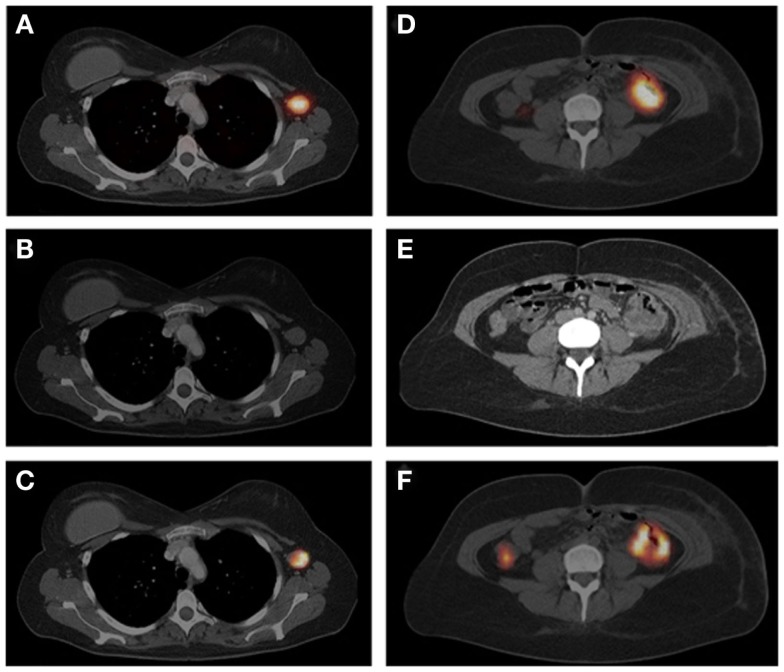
The SPECT/CT image (A), acquired 24 h after injection of ^111^In-IMP288 (185 MBq, 25 μg), pretargeted with 75 mg TF2 (1-day interval), in a 38-year-old patient (cohort 4), shows very clear tumor targeting of an axillary lymph-node metastasis, with very low concentrations of radioactivity in normal tissues. Corresponding contrast-enhanced CT scan and a fused FDG-PET/CT scan are shown [(B,C), respectively]. The primary colon tumor (50 cm ab ano) also shows highly specific tumor targeting in the SPECT image (D), confirmed by the CT scan and FDG-PET/CT [(E,F), respectively], with non-specific FDG uptake in the ascending colon. This research was originally published in Schoffelen et al. (52).
